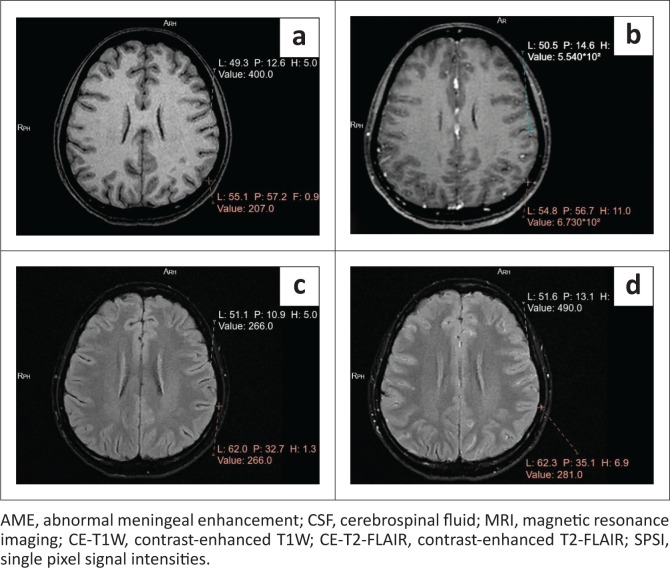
Axial brain MRI of a 22-year-old female patient with CSF proven meningitis showing placement of the region of interest (ROI) and measurement of SPSI. The values in white represent meningeal SPSI and the values in red represent vascular SPSI. (a) Pre-contrast T1W image. (b) CE-T1W image where AME is inconspicuous. Single pixel signal intensities of the meninges (554) are less than the SPSI of the vessels (673). (c) Pre-contrast T2-FLAIR image. (d) CE-T2-FLAIR where AME is clearly visualised. Single pixel signal intensities of the meninges (490) are higher than the SPSI of the vessels (281).
